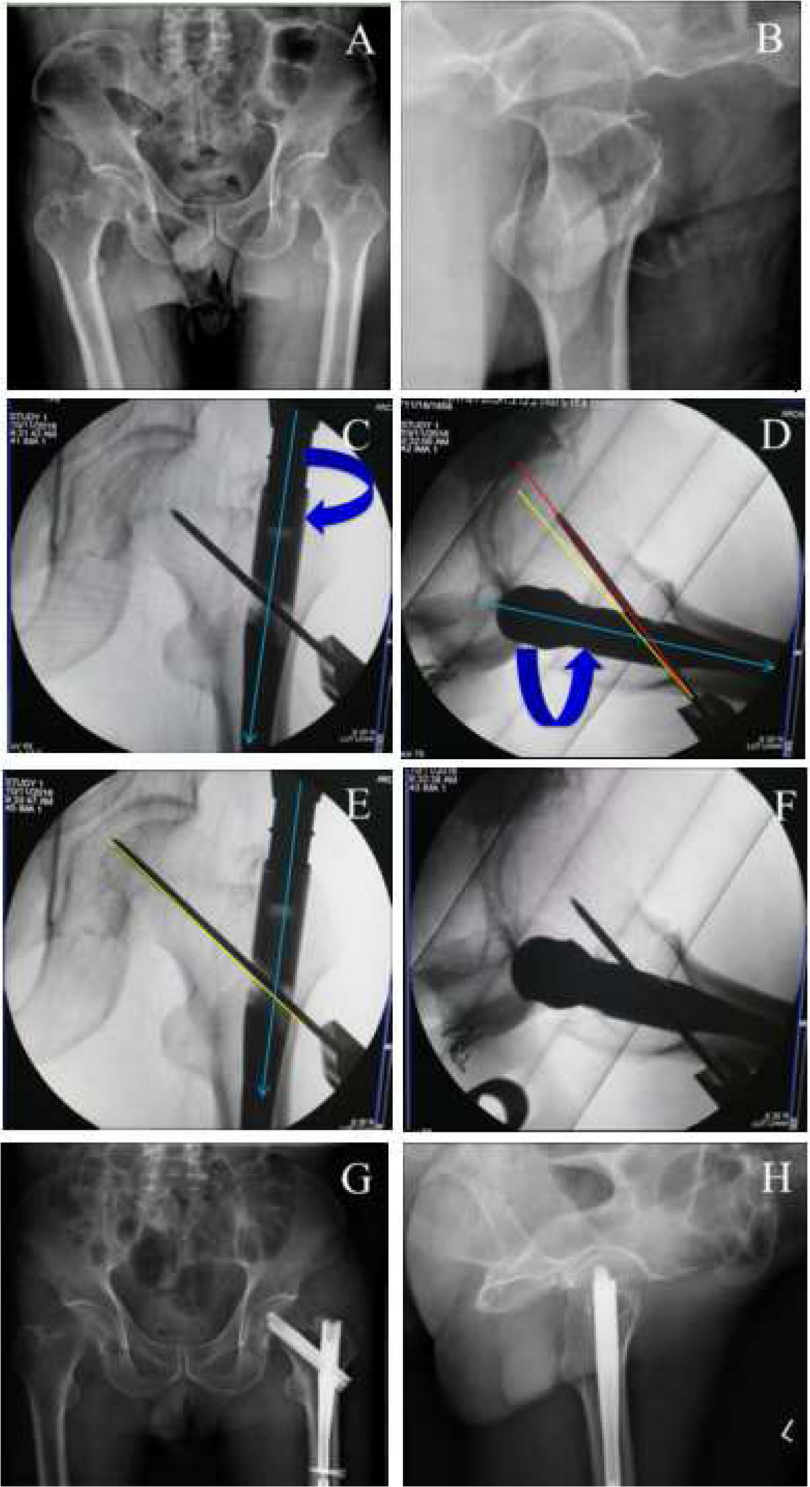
A typical case to regulate the PSA and anteversion angle to obtain ideal TAD. a-b, preoperative anteroposterior (a) and lateral (b) X-ray to diagnose intertaochanteric fractures; c-f, the adjustment of the guide pin. When position of neck guide pin is higher (the PSA is larger, c) and anteversion angle of nail is more than normal (d), we need to internally rotate the nail to make anteversion angle smaller (f) (at the same time, the PSA will become less in AP view, e) to get better TAD; g-h, postoperative anteroposterior (g) and lateral (h) X-ray to confirm the fracture reduction. Green line, nail position; red line, current position; yellow, ideal position
